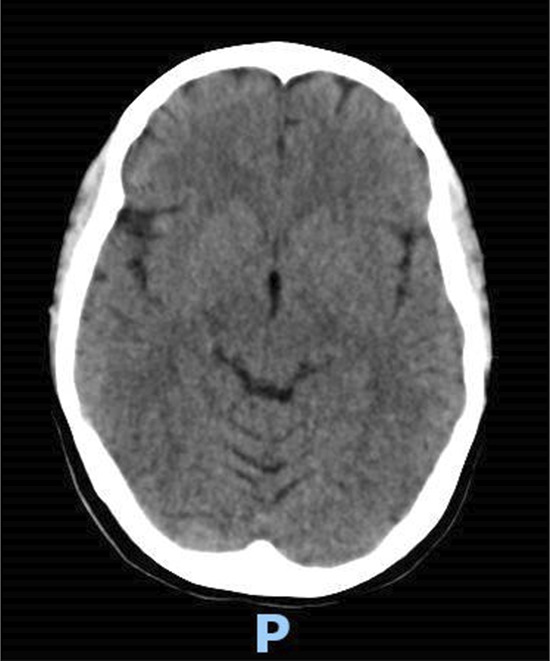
Brain CT scan without contrast showed no hemorrhage; normal brain CT. CT, computed tomography.
